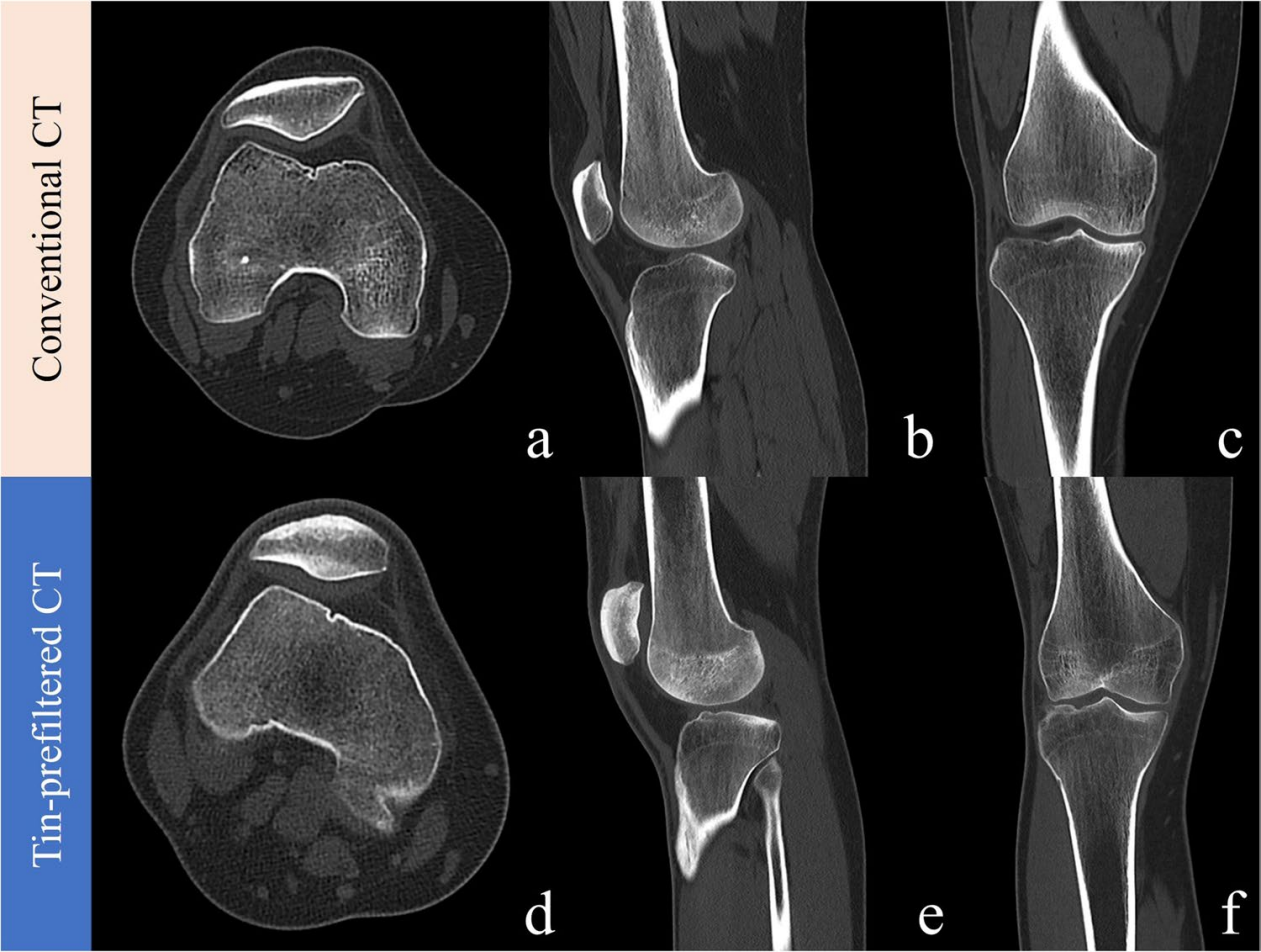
Conventional CT scan of the knee of a 26-year-old female (BMI 25.0 kg/m^2^) (a, b, c) compared to a tin-prefiltered CT scan of the knee of a 22-year-old man (BMI 24.9 kg/m^2^) (d, e, f). CTDI_vol_ of the tin-prefiltered scan was 2.59 mGy compared to 9.06 mGy of the conventional CT scan. All images were reconstructed with the convolution kernel BR59, iterative reconstruction strength level 3, and displayed at a window/center level of 1500/450. Axial images were reconstructed with a slice thickness of 1 mm, sagittal and coronal images at 2 mm slice thickness
